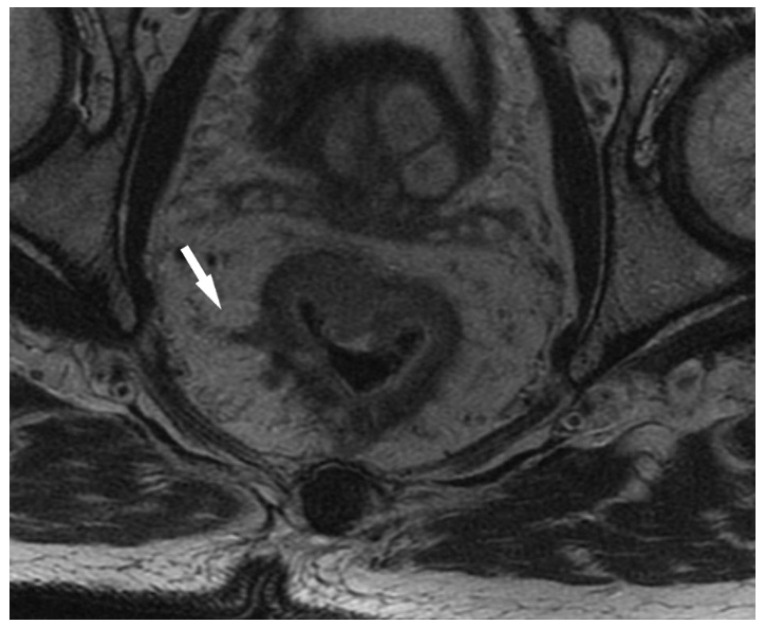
EMVI-positive rectal cancer. Axial T2-weighted MRI scan showing EMVI (white arrow).
